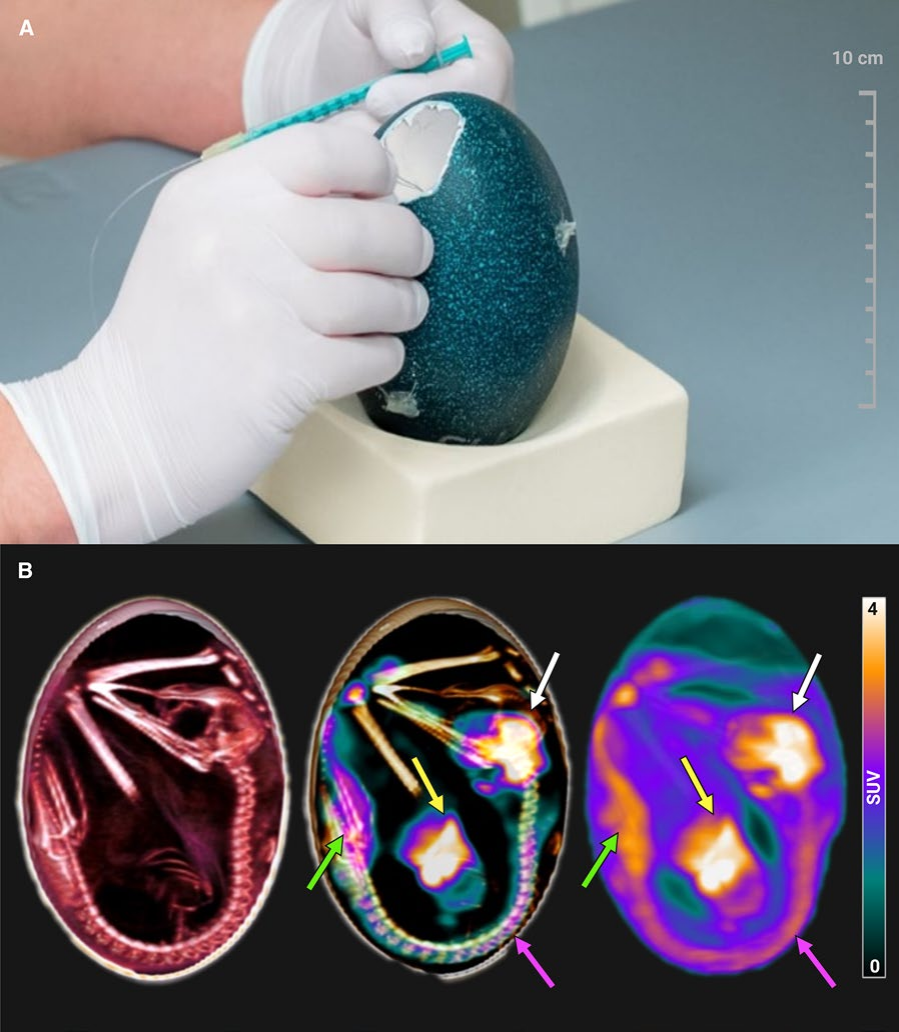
(A) Preparation for intravenous administration of [^18^F]FDG. (B) Representative PET/CT with typical anatomical (left side), metabolic (right side), and fusion images (middle). [^18^F]FDG uptake was evident in brain (white arrows), liver (yellow arrows), kidneys (green arrows), and spinal cord (pink arrows) (Created in https://BioRender.com). Additional visualizations are shown in Supplementary material 4, 5, and 6
